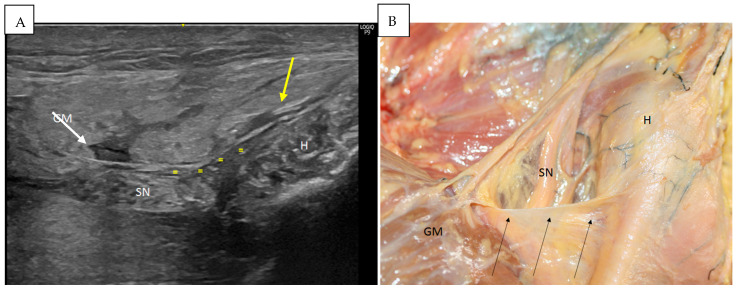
(A) The expansion over the sciatic nerve (SN) on the left side. An ultrasound study allows us to visualize this expansion (yellow =), which goes from the origin of the hamstrings (H) (yellow arrow) to the gluteus maximus muscle (GM) (white arrow). IT: Ischial tuberosity. (B) Anatomical study allowing us to see this expansion (black arrows) over the sciatic nerve on the left side. It is possible to visualize that it goes from the origin of the hamstrings (H) (yellow arrow) to the gluteus maximus muscle (GM) (white arrow).
